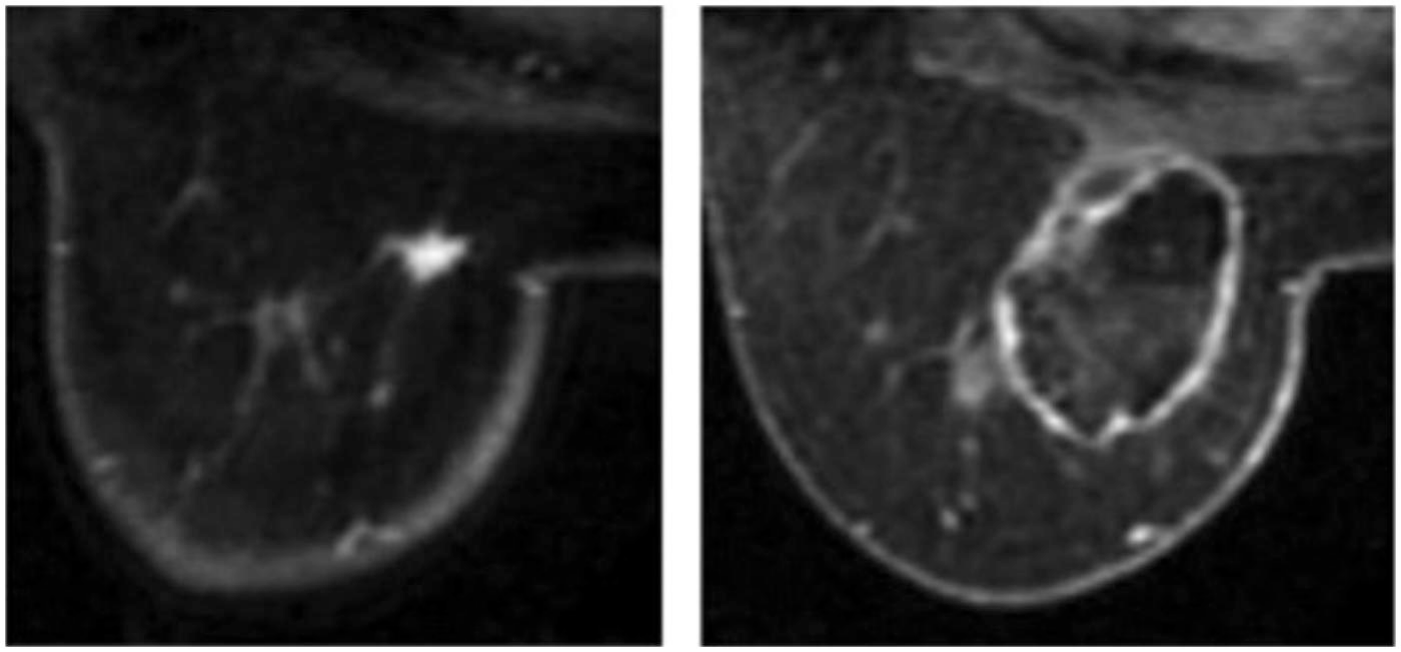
(Left) MRI before RFA; (Right) MRI after RFA.^34^
